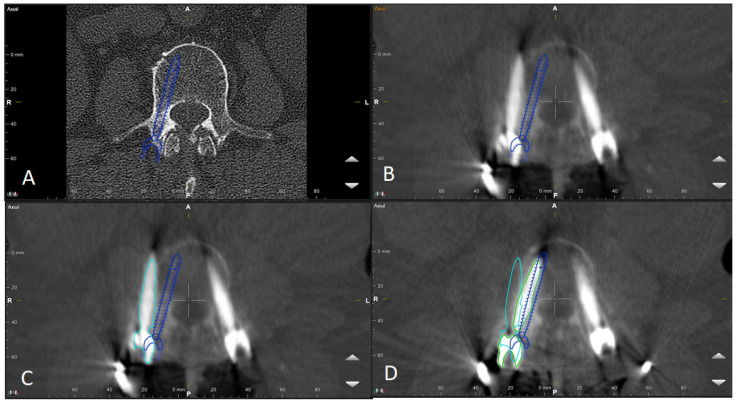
Strategies in visualizing an incorrectly placed screw. (A) Visualization of the preplanned screw in the preoperative CT scan. (B) iCT to check the screw position shows misplacement of the screw (GRS E) with visualization of preplanned screw. (C) Segmentation of the incorrectly placed screw in iCT. (D) Control iCT scan with correct position of the screw along the preplanned trajectory and the position of the originally incorrectly placed screw.
